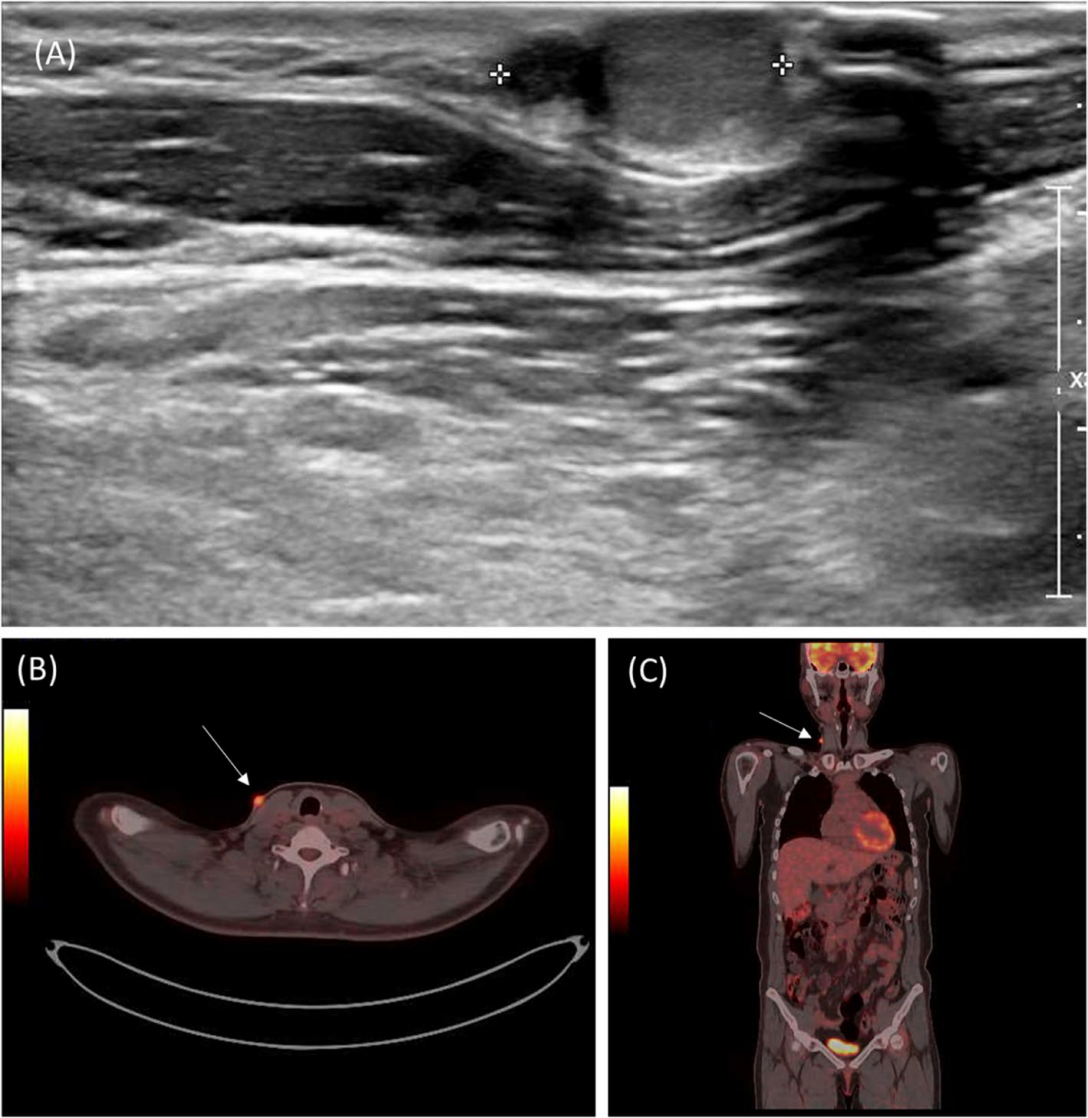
A Preoperative ultrasound of the right neck skin lesion. B and C PET/CT showed a hypermetabolic lesion in the right neck and no other abnormal hypermetabolic lesions
